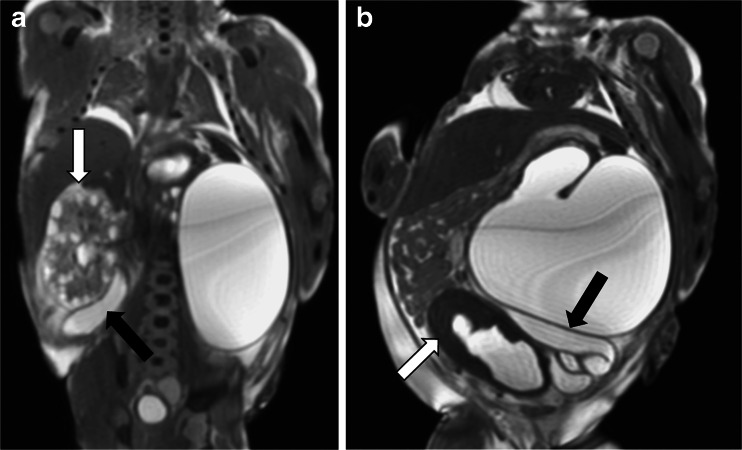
Postmortem MRI of the abdomen in a female fetus of 22 weeks gestation. Coronal (a) and parasagittal (b) T_2_-weighted postmortem MR images in a late stillbirth with obstructive uropathy. The right kidney is multicystic dysplastic (*white arrow* in a) and the left kidney is obstructed, with gross tortuous dilatation of both ureters (*black arrows*), and a thickened trabeculated bladder (*white arrow* in b), representing bladder outflow obstruction. Secondary pulmonary hypoplasia is also demonstrated. Posterior urethral valves were confirmed at autopsy
